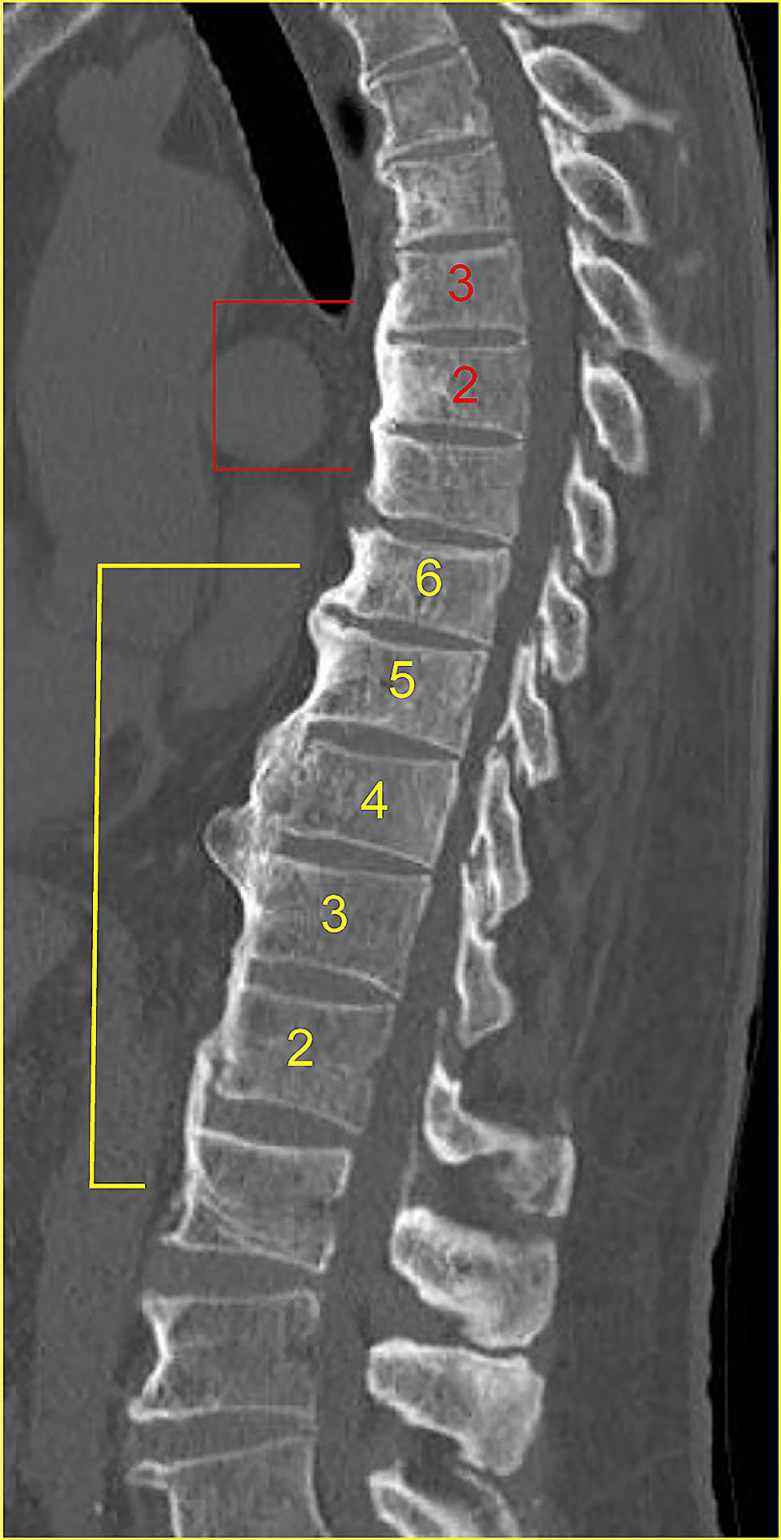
Calculation of maxVB. Sagittal CT image spanning the thoracic vertebra to the lumbar vertebra showing continuous bony bridges between adjacent vertebrae at vertebrae 3 and 6. The maximum number of contiguous bone bridges between adjacent vertebrae was defined as the maxVB, which was 6. Abbreviations: maxVB, maximum number of vertebral bodies that are bony cross-linked with contiguous adjacent vertebrae
